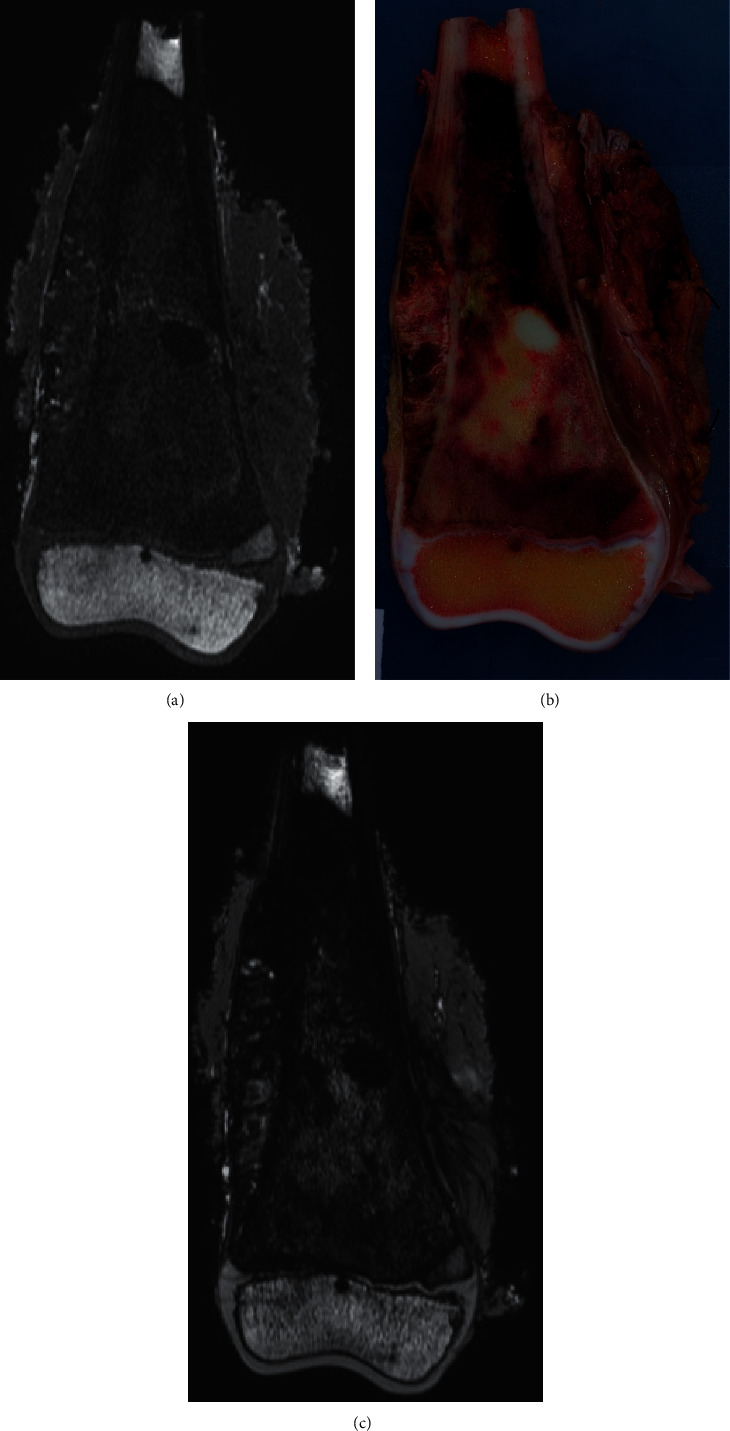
An 11-year-old boy with distal femur osteosarcoma. No PSI was used because 17.5 cm bone resection was necessary for a growth prosthesis. One margin was measured. (a) T1-weighted MRI; (b) macroscopic cut; (c) T2-weighted MRI.
